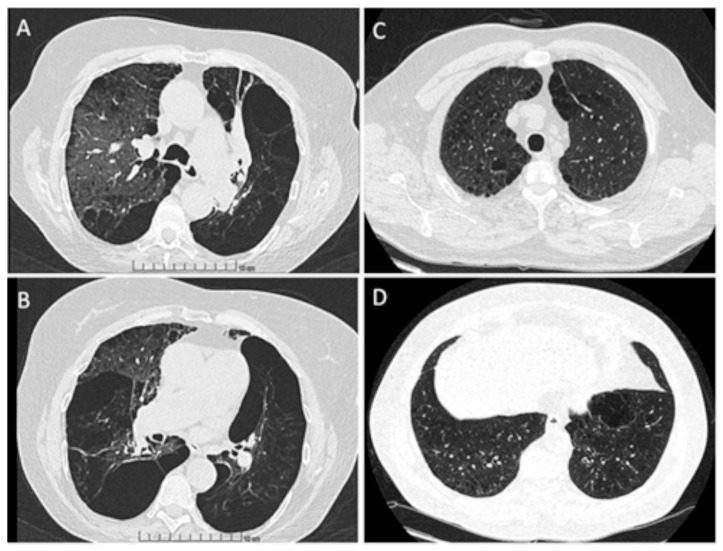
Chest high-resolution CT scan; axial view of upper lobes (A) and lower lobes (B) of lungs of an active smoking patient with M/MProcida genotype; axial view of upper lobes (C) and lower lobes (D) of lungs of a never smoker patient with M/I genotype.
